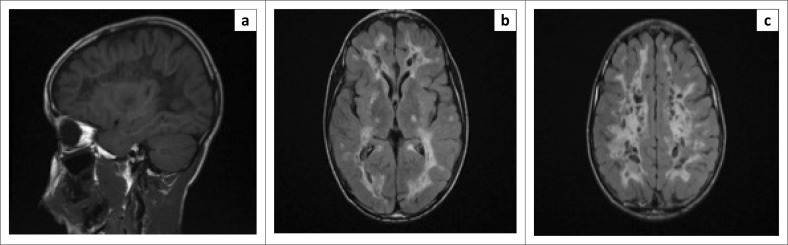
Magnetic resonance imaging after 1 year: (a) parasagittal T1 weighted imaging (T1WI) demonstrates radiating stripes stretching across the rarefied white matter, which is suggestive of the remaining normal white matter tracts. (b and c) Axial fluid attenuation inversion recovery (FLAIR) images with significant progression in the white matter signal abnormality and cystic degeneration.
